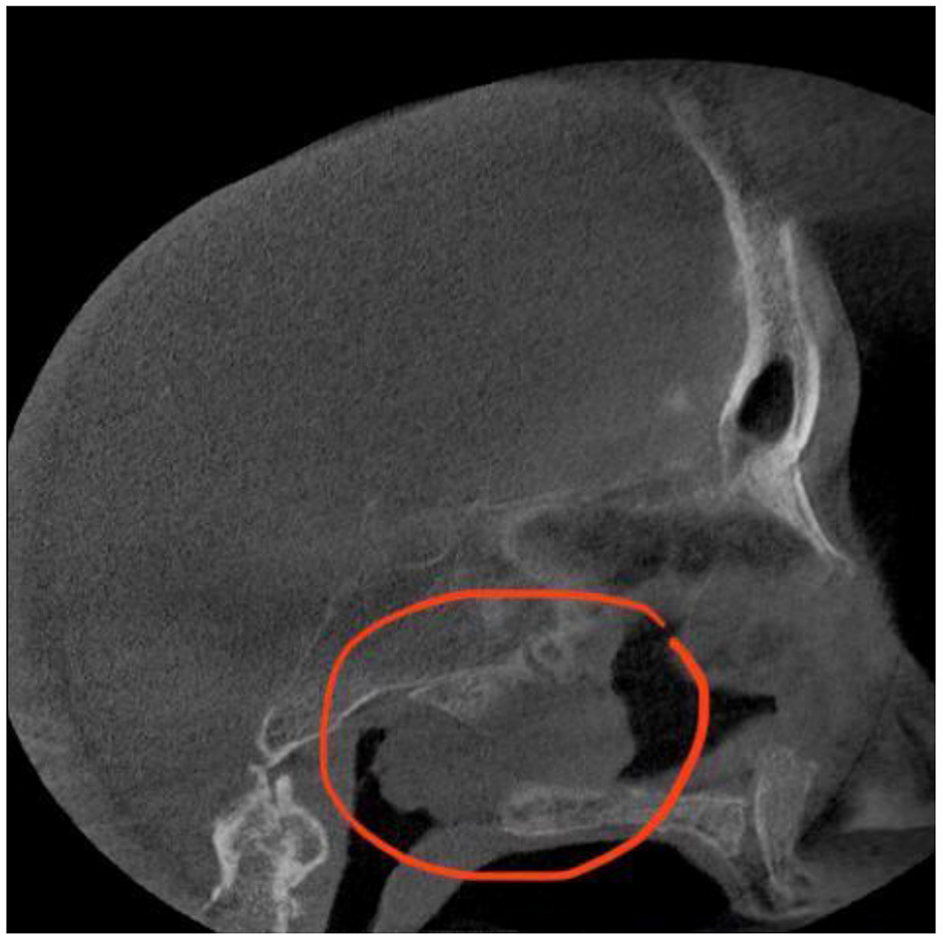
Pre-operative CT scan sagittal view.
